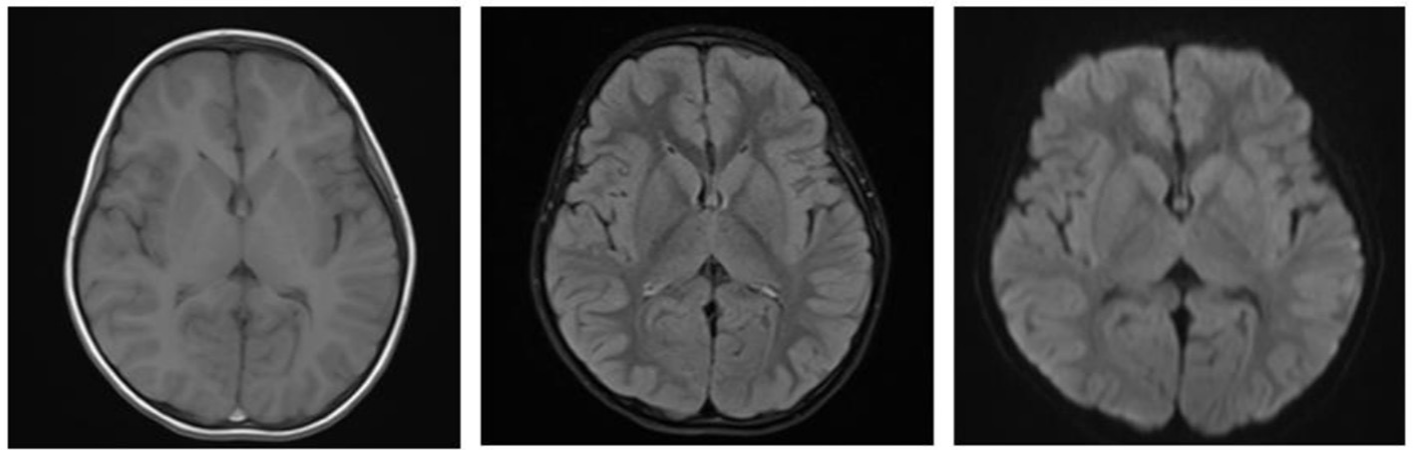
Cranial MRI on admission.
